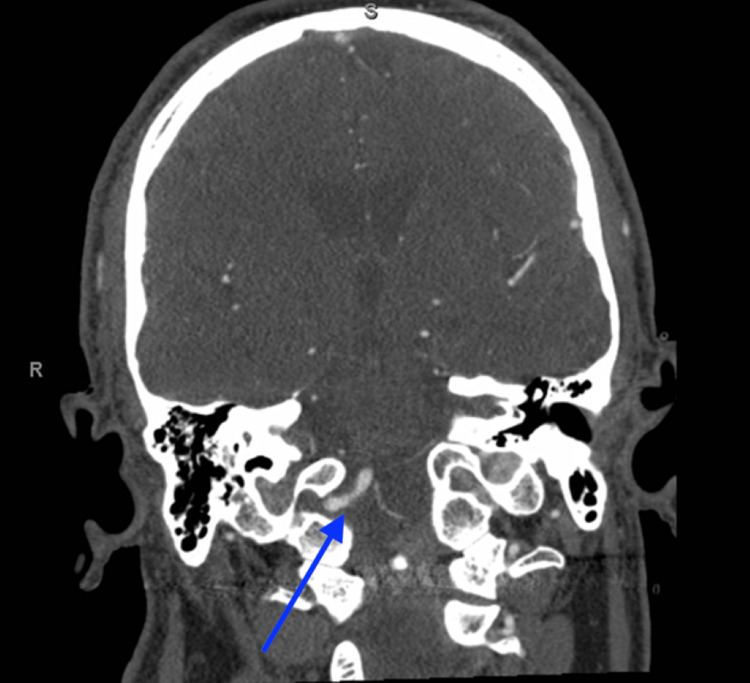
Computed Tomography Angiography Depicting the Right PPHA in Coronal View
